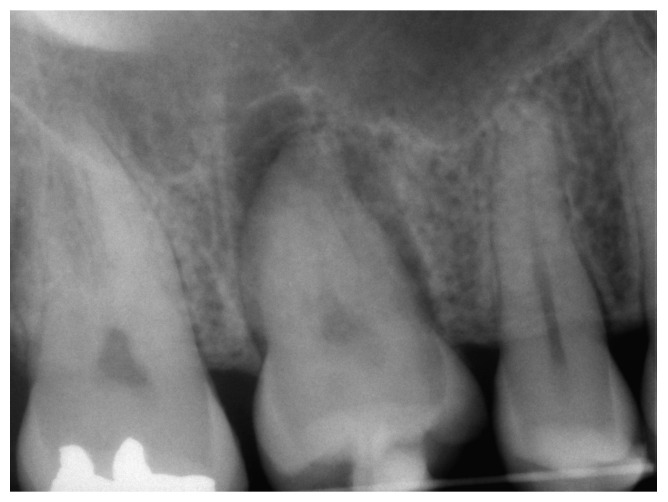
Post-operative periapical X-ray.
